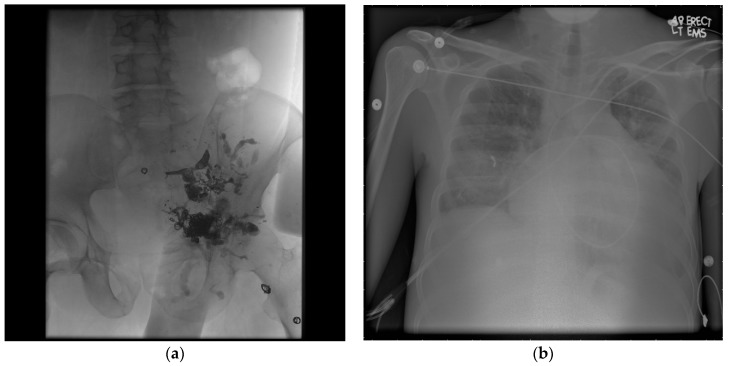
Schobinger Stage IV arteriovenous malformations (AVM). (a) The post-treatment angiogram shows the extent of a dilated hypogastric venous system due to drainage from the nidus and presence of a pelvic AVM with multiple outflowing veins as well as one particularly large flowing vein. The AVM has been treated from the venous side with coils and from the arterial side with a liquid embolic agent, indicative of a possible Yakes IIb/IIIb angiographic type. (b) A chest X-ray shows that the patient also presented with cardiomegaly and high-output cardiac failure due to stress on the venous circulation.
